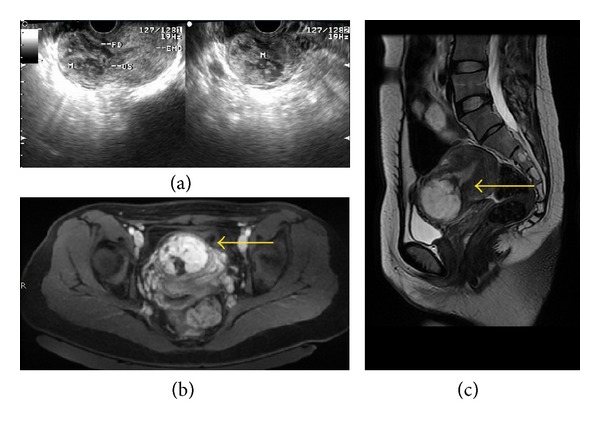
(a) Transvaginal gray-scale ultrasonography revealing an irregular gestational cystic mass (FD) located within the isthmic area of the lower anterior wall of the uterus and protruding toward the uterine cavity. (b) Obvious and homogenous enhancement of the gestational sac after Gd-DTPA administration on axial T1-weighted imaging (arrow) without enhancement of the inner content. (c) Huge gestational sac (4.9 cm × 4.0 cm × 4.4 cm) showing high signal intensity on sagittal T2-weighted imaging (arrow) with low signal intensity inside.
